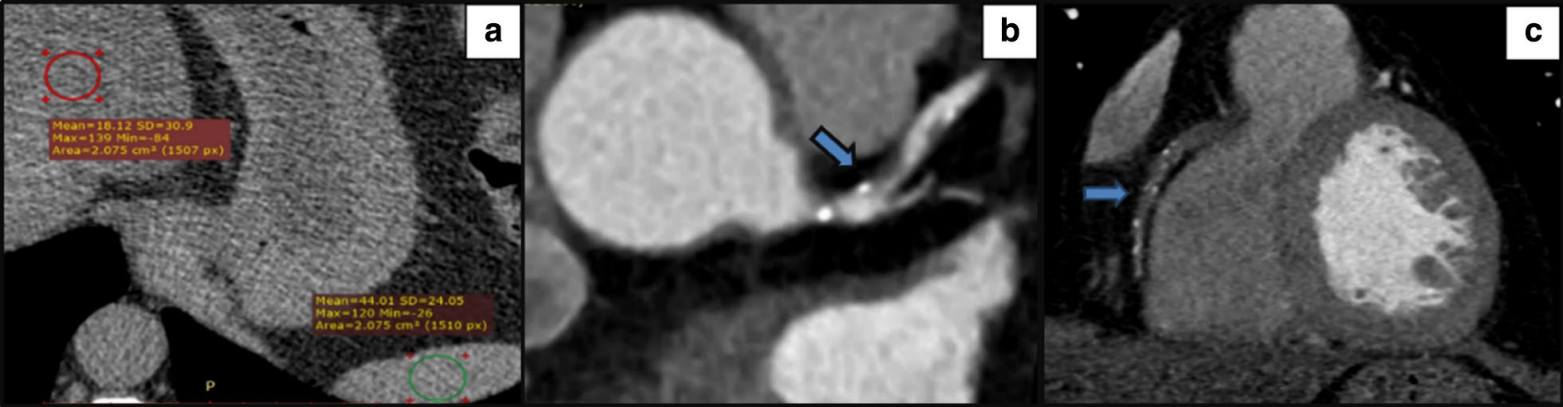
Hypertensive male patient 55 years old. a Axial non-enhanced CT image demonstrates attenuation measurements of the liver and spleen showing fat accumulation in the liver, with a mean liver attenuation of 18.1 HU and a mean spleen attenuation of 44 HU. b Coronary CT angiography shows partially calcified plaque with a low CT number (17 HU) (arrow) and positive remodelling and c Coronary CT angiography shows totally occluded RCA with multiple spotty calcifications (arrow)
